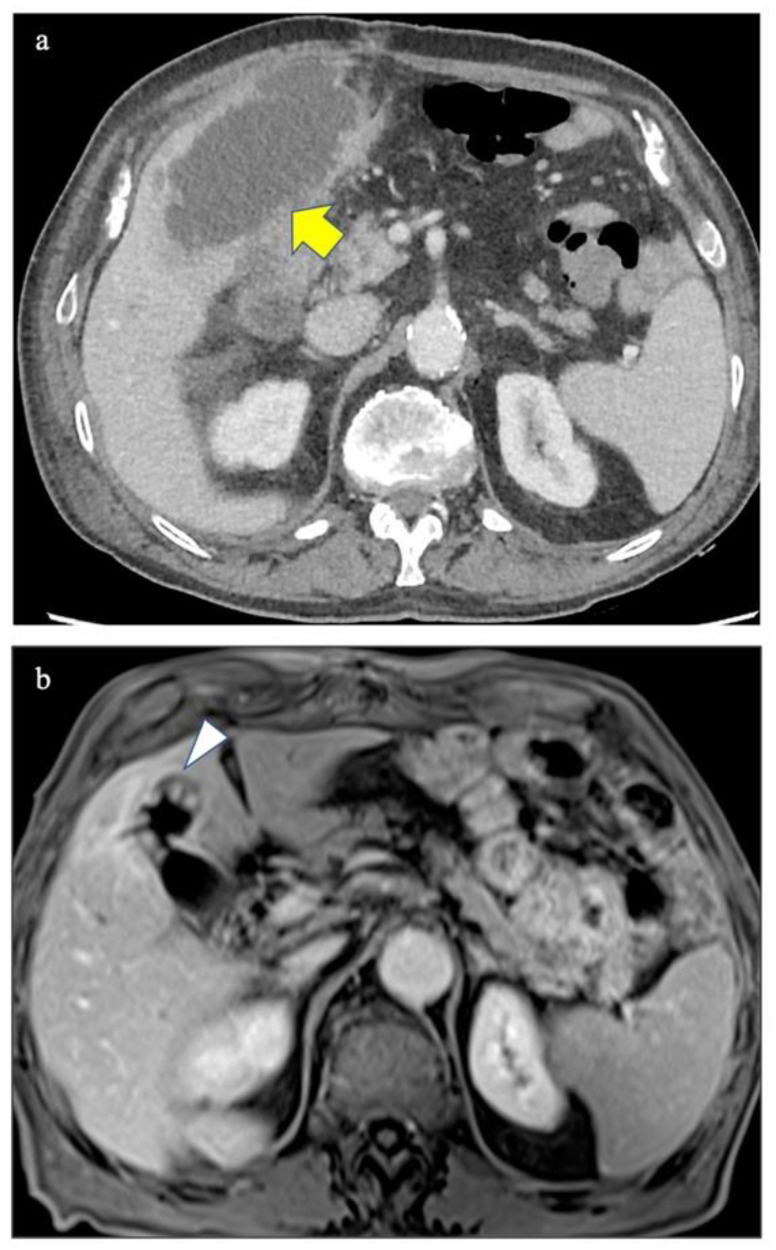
Axial contrast-enhanced Computed Tomography image of the upper abdomen well-demonstrating the acute cholecystitis with localized perforation (yellow arrow in (a)) and fat-saturated Volume Interpolated Breath-Hold Examination (VIBE) Magnetic Resonance image after contrast injection performed 90 days later showing multiple small pseudopolyps in the fundus of the gallbladder (white arrow in (b)).
